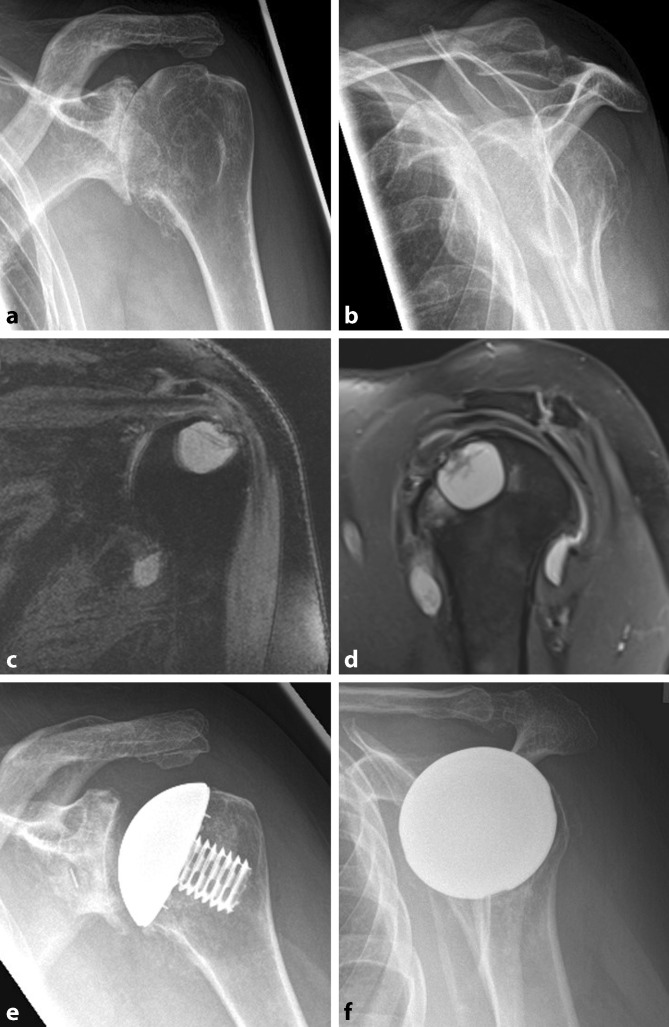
a, b Conventional radiographic imaging of a left shoulder revealed severe primary osteoarthritis with extensive humeral and glenoidal osteophyte formation. c, d Further magnetic resonance imaging of the affected shoulder showed a large bone cyst at the posterosuperior portion of the proximal humerus, yet the rotator cuff was figured to be intact. e, f Postoperative radiographic imaging after total shoulder arthroplasty with a stemless humeral component and humeral autograft
